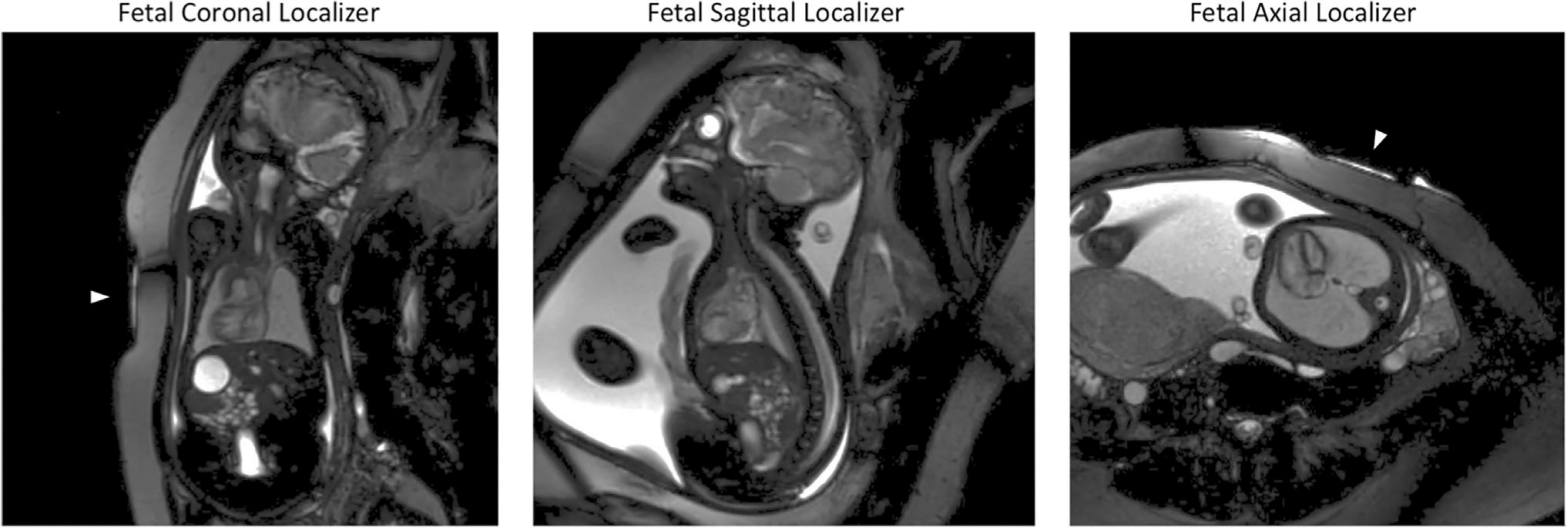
Presented are three planning localizers in fetal coronal, sagittal and axial orientation obtained at 1.5 T MRI in a fetus at gestational age of 36 weeks. Arrowhead shows the position of the Doppler ultrasound probe and the hyperintense signal from the ultrasound gel. Figure courtesy of Erin Englund and Alex J. Barker (Department of Radiology, Pediatric Radiology University of Colorado, Anschutz Medical Campus, USA)
